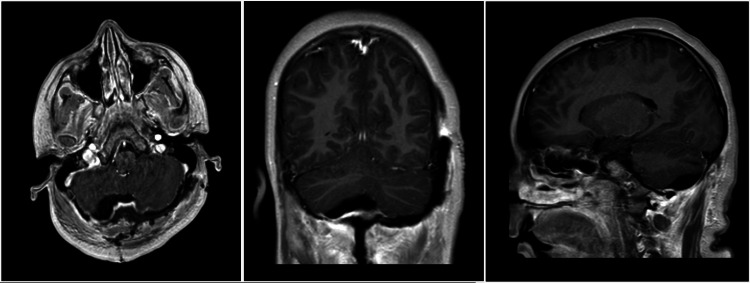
Post-operative MRI Postoperative magnetic resonance imaging with contrast reveals gross total resection of the right occipital calvarial lesion.
